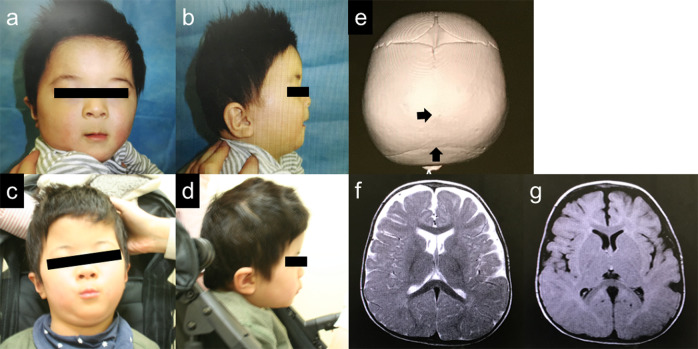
Clinical information of the patient. Facial appearance of the patient at 6 months of age (a, b) and 5 years of age (c, d), suggesting deformity of the skull, forehead protrusion, arched eyebrows, flat nose base, thin upper lip, small mouth, long philtrum, retrognathia and low-set ears. e 3-dimensional CT image of the skull at 10 months of age shows partial early fusion of the sagittal and lambda sutures (arrows). T1-(f) and T2-(g)-weighted brain MRI at 11 months of age showed no apparent abnormalities.
